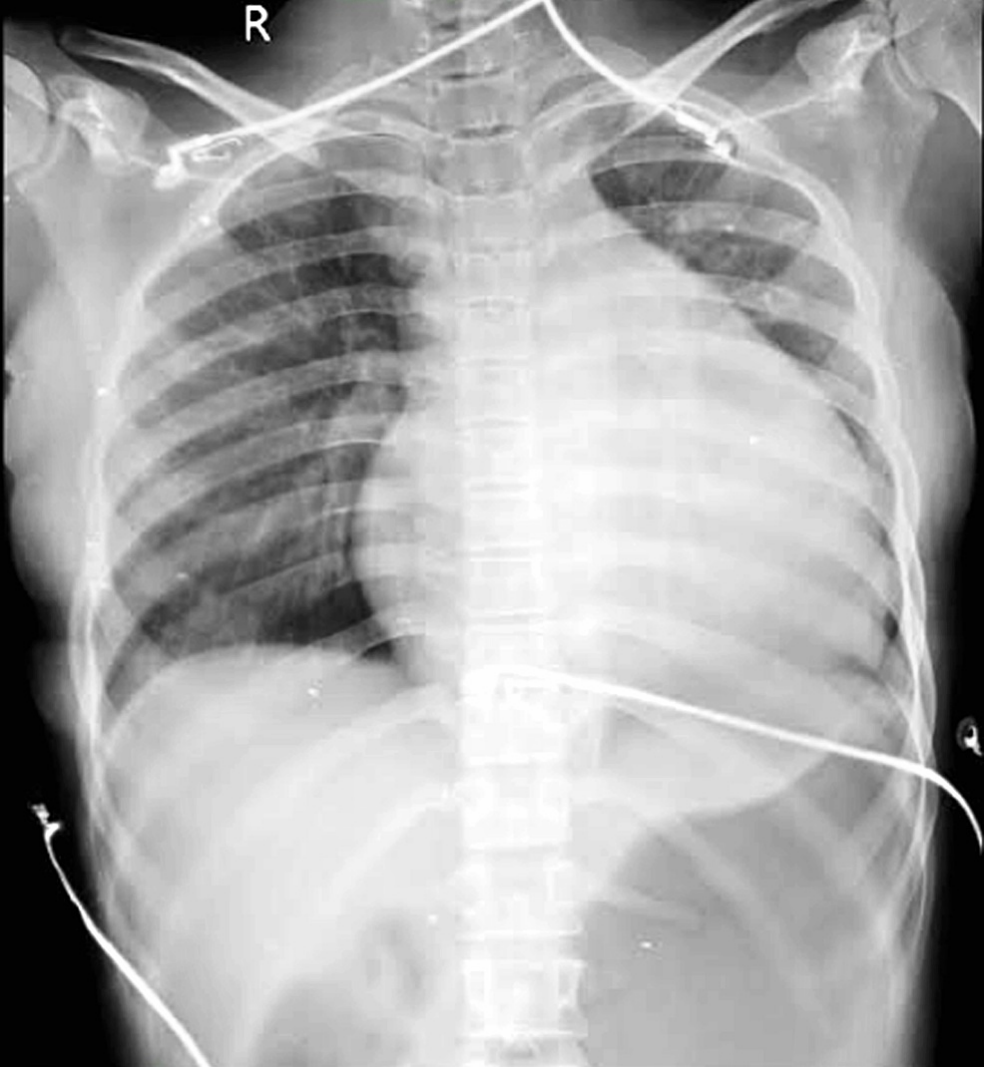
Chest X-ray of patient showing cardiomegaly
